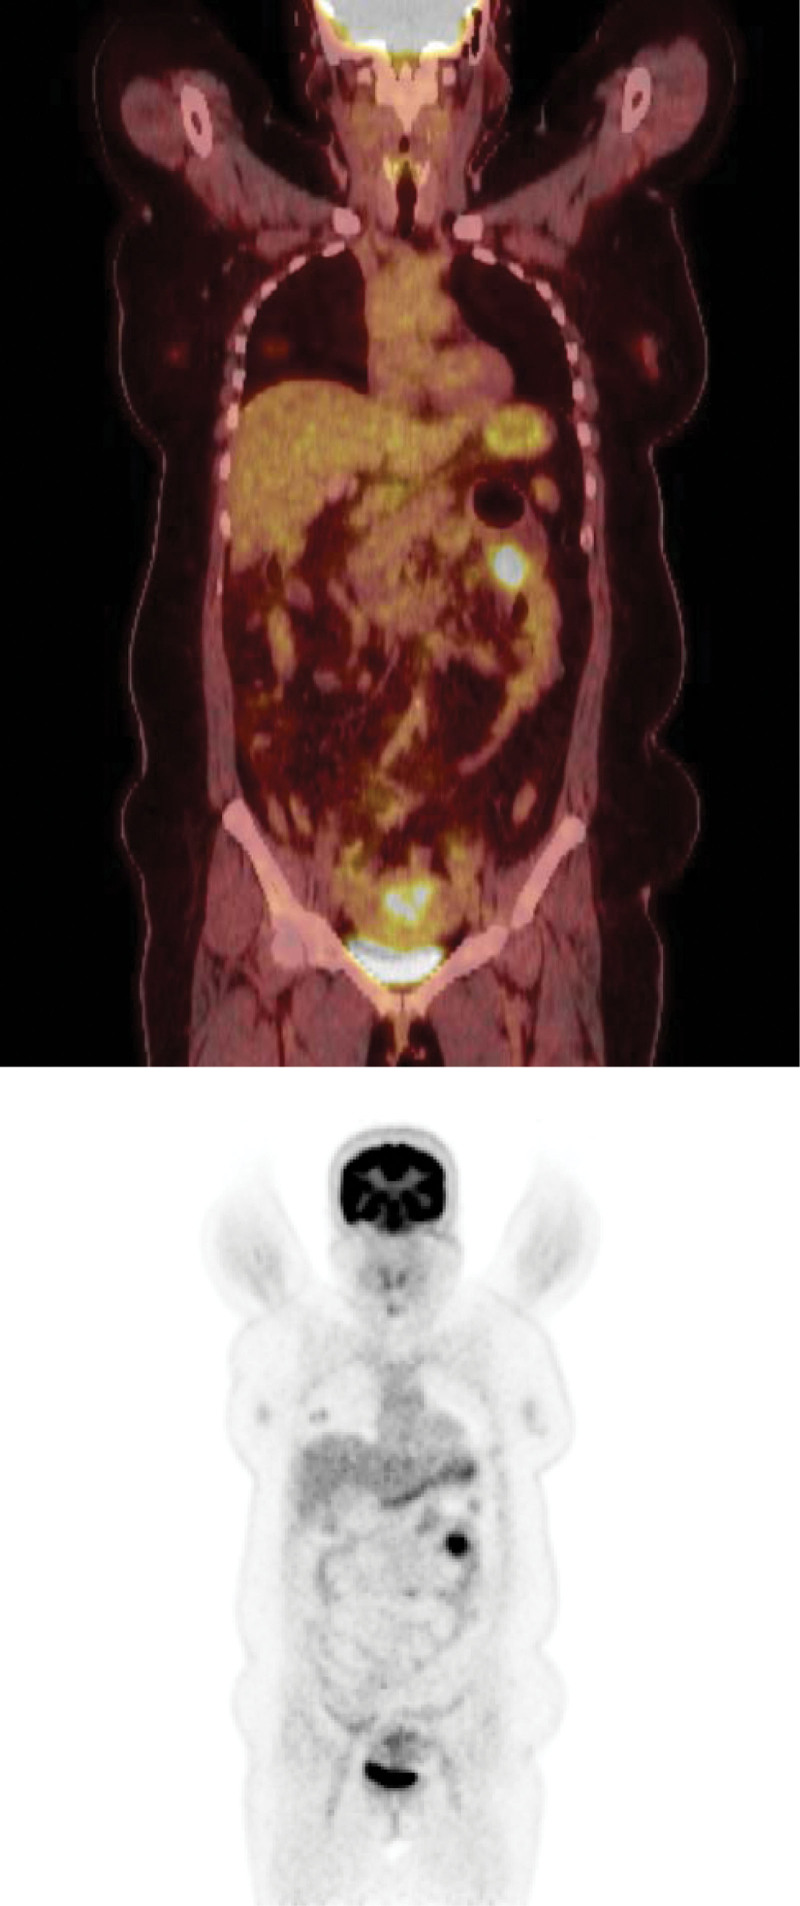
Coronal 18F-FDG PET CT Images. PET CT = Fluorine-18-fluorodeoxyglucose positron emission tomography.
